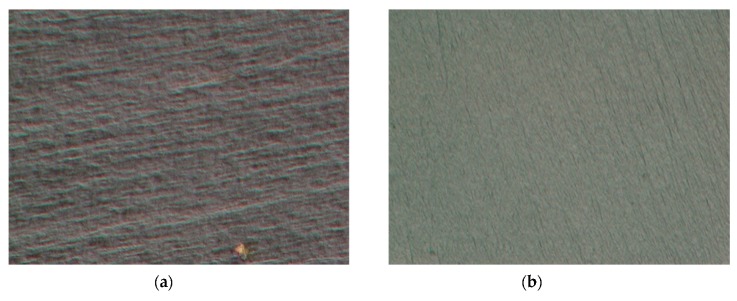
The fracture surfaces of neat DCPD with decelerator under CT (−160 °C) condition. ((a) 1 wt.% decelerator, (b) 2 wt.% decelerator, up to 2000× magnification).
